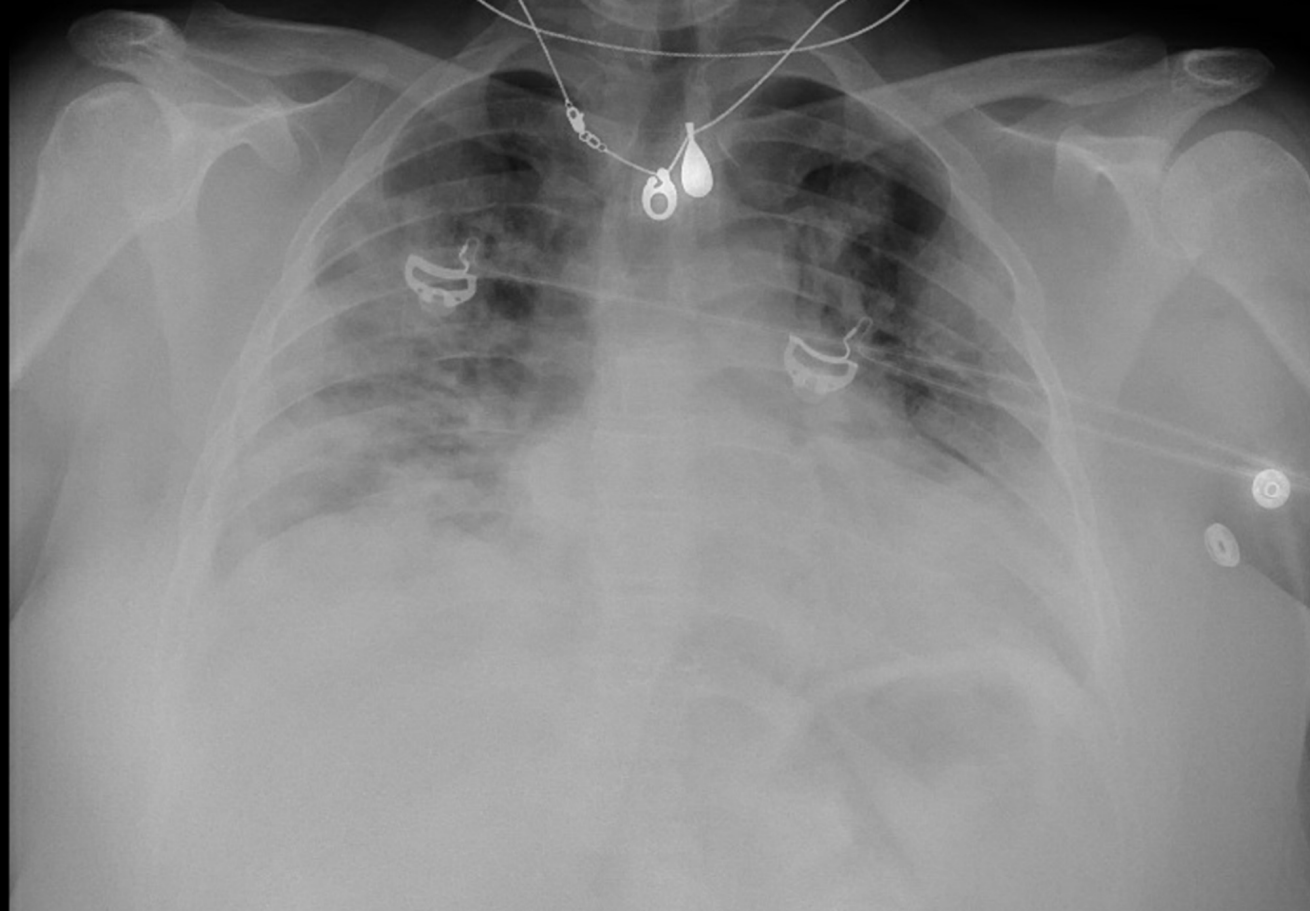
Chest radiograph Chest radiograph from admission was consistent with COVID-19 pneumonia, with multiple rounded and peripheral ground-glass opacities throughout the lung fields.
